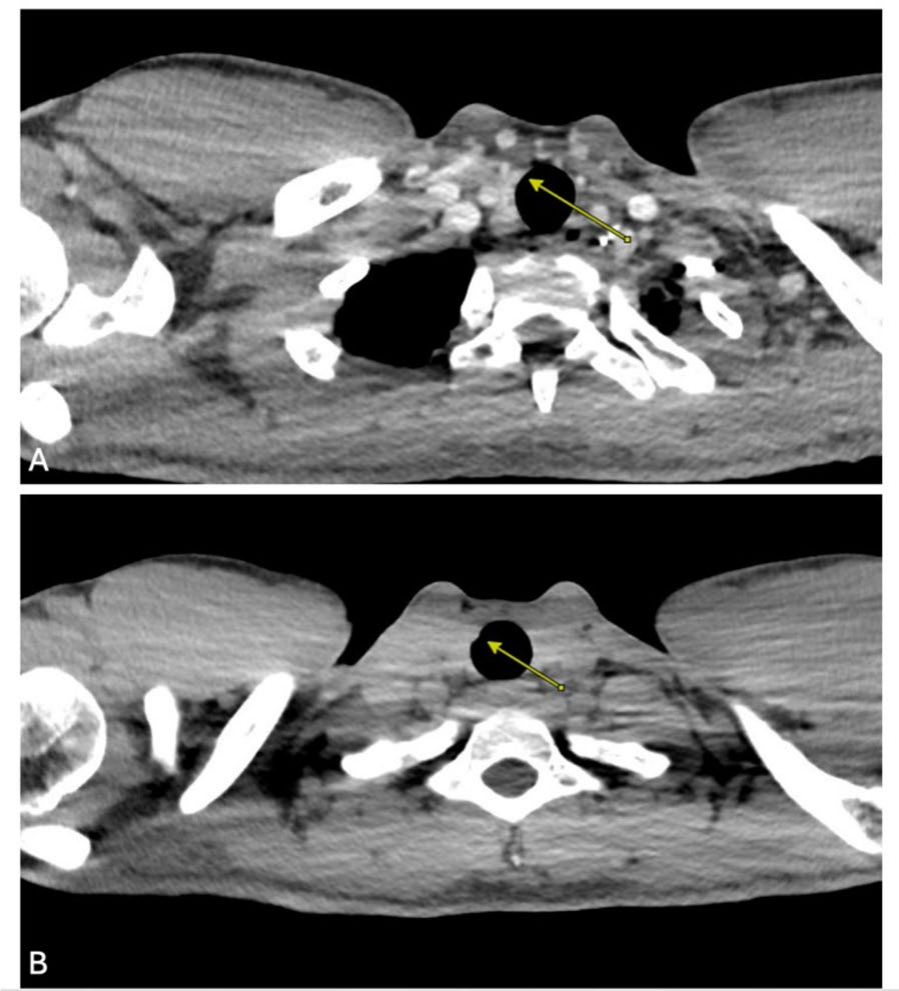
A Preoperative computed tomography with contrast chest showing tracheal nodularity. B A total of 1 year post-treatment, demonstrating persistent nodularity. Yellow arrow denotes area with persistant nodularity
